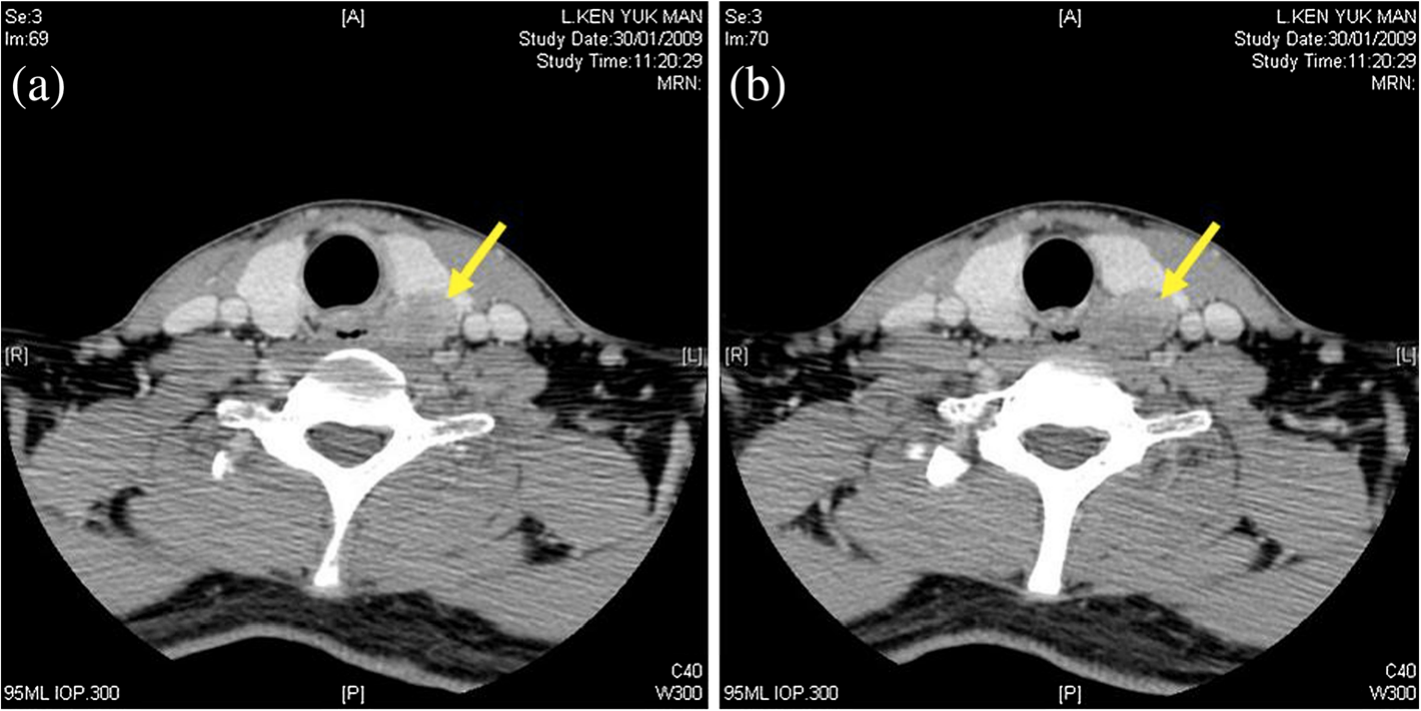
(a,b). Axial computed tomography scan of the neck. A hypodense lesion 30 × 20 × 20 mm in size was visible in the posterior thyroid (arrow) without cervical lymphadenopathy or local infiltration.
